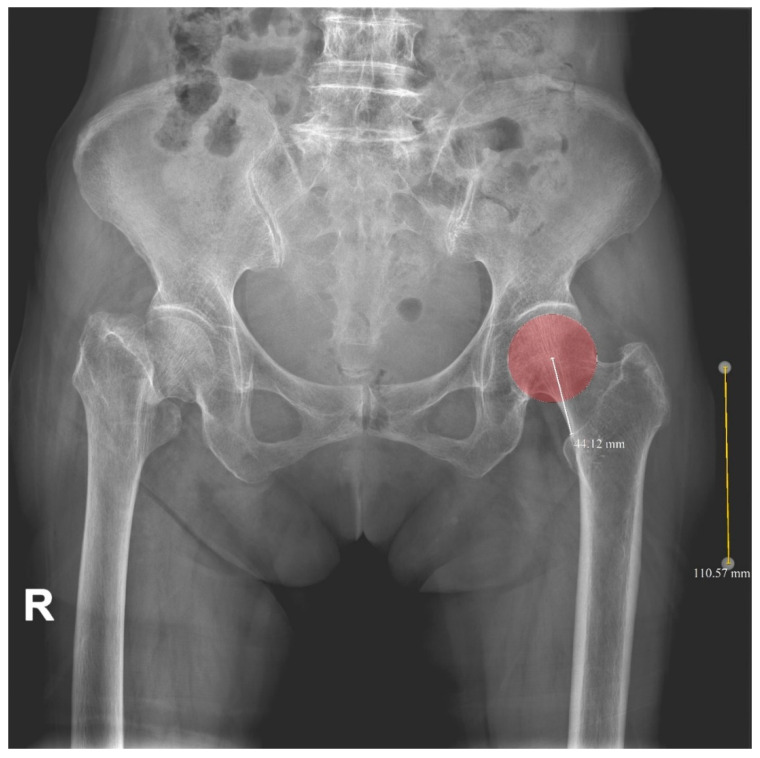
Two-dimensional head-lesser trochanter distance (2-D HLD). Magnification was corrected through a 10-cm sized magnification marker. R: right-sided on radiographs.
